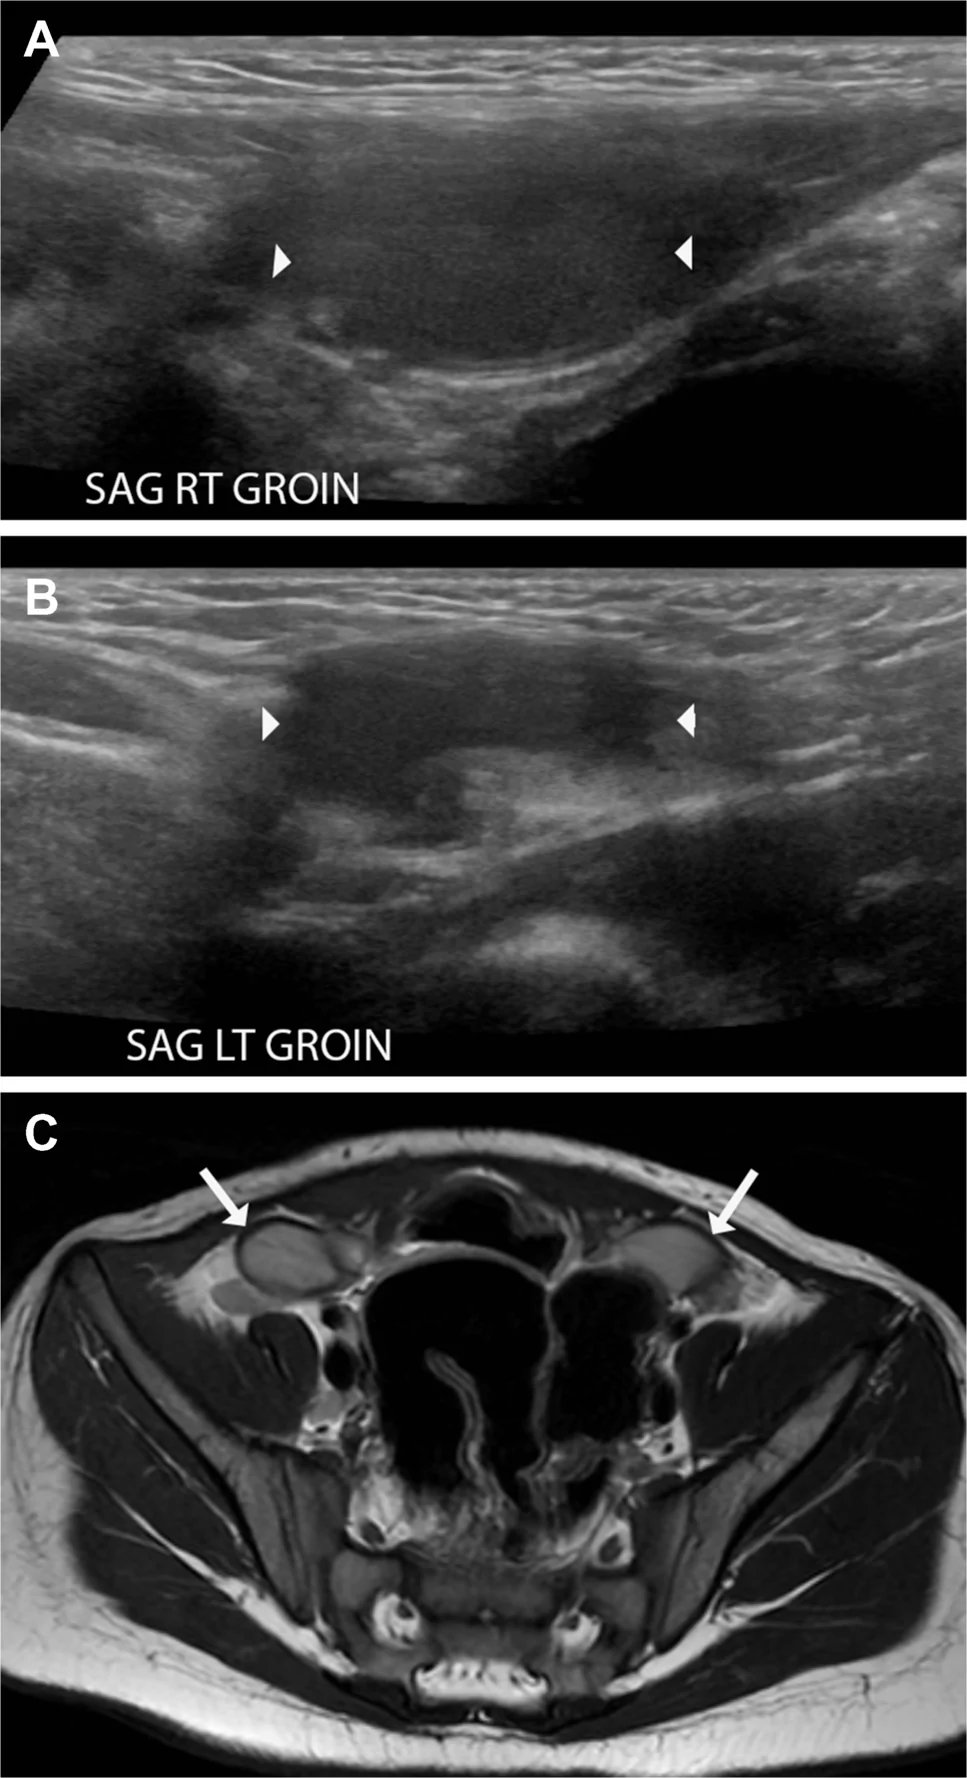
Androgen insensitivity syndrome suspected in a 1-year-old 46,XY infant with normal external female genitalia. Pelvis ultrasound images of the (a) right and (b) left inguinal regions show oblong homogenous solid tissue within the bilateral inguinal canals most consistent with testicles (*arrowheads*). c The gonads resemble testicles in lower pelvis (*arrows*) on subsequent T2-weighted MRI acquired 2 months later. Renal ultrasound shows normal appearances of bilateral adrenal glands and kidneys (*not shown*). No discernible ovaries or uterus was identified by ultrasound or MRI (*not shown*)
